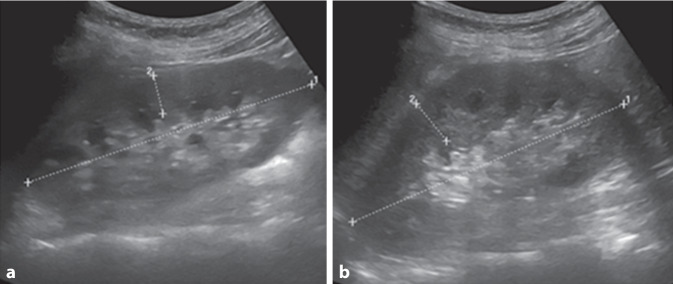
Sonographisch orthotope Lage und regelhafte Größe der Nieren beidseits, a r*echts* 11,6 cm lang, b *links* 11 cm, mit beidseits regelhafter Parenchymdicke, a *rechts* 1,59 cm, b *links* 1,91 cm, und kleine Konkremente im Nierenbeckenkelchsystem (Nierengrieß) beidseits ohne Harnstauung
